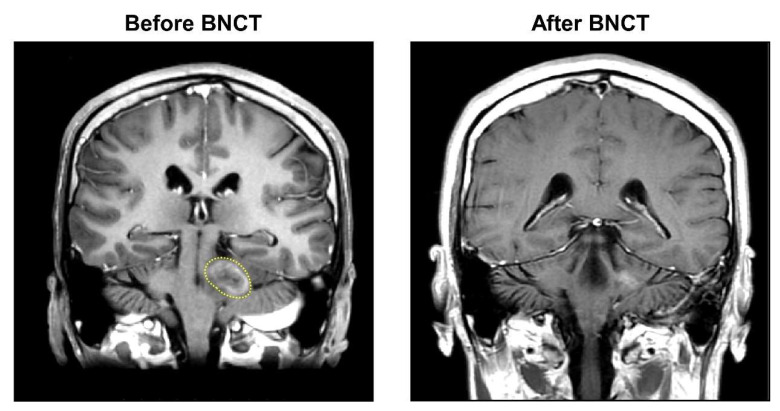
MRI images of tumor shrinkage of Case 3 (patient #7). After a scheduled two fractions of salvage BNCT procedures, the patient achieved a CR with obvious tumor shrinkage. The yellow dashed line marks the malignant glioblastoma.
